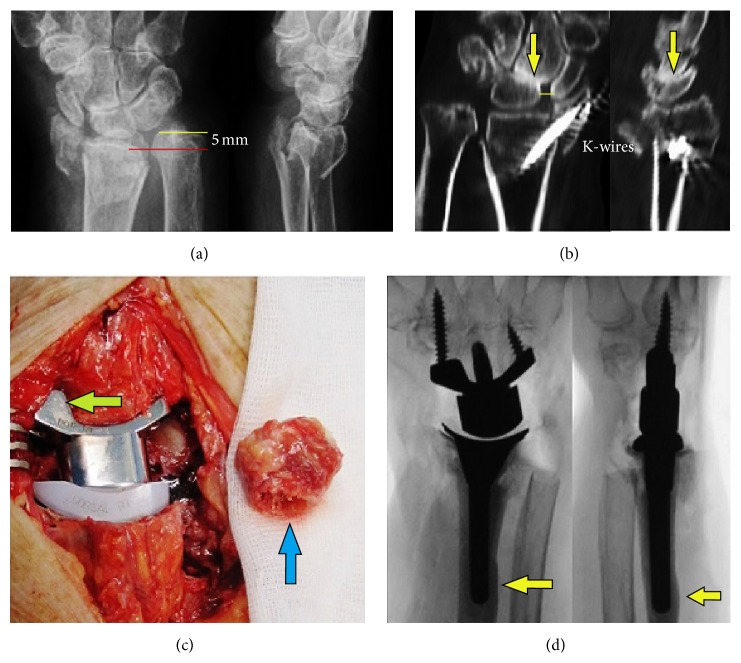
Case report 2 (preoperative and surgical procedures): (a) initial AP and lateral radiographs; (b) coronary and sagittal CT images after removal of external fixateur and percutaneous pinning demonstrating a step-off in the radial articular surface of 3 mm and widened scapholunate gap (*line*) with midcarpal OA (*arrow*); (c) intraoperative clinical photo after Maestro WRS insertion by using a scaphoid augment of the carpal component after complete scaphoid excision (*arrow*) and resected ulnar head (*arrow*); (d) intraoperative AP and lateral fluoroscopy showing correct position of implant and the leakage of cement from one fixateur pin-hole (*arrows*).
